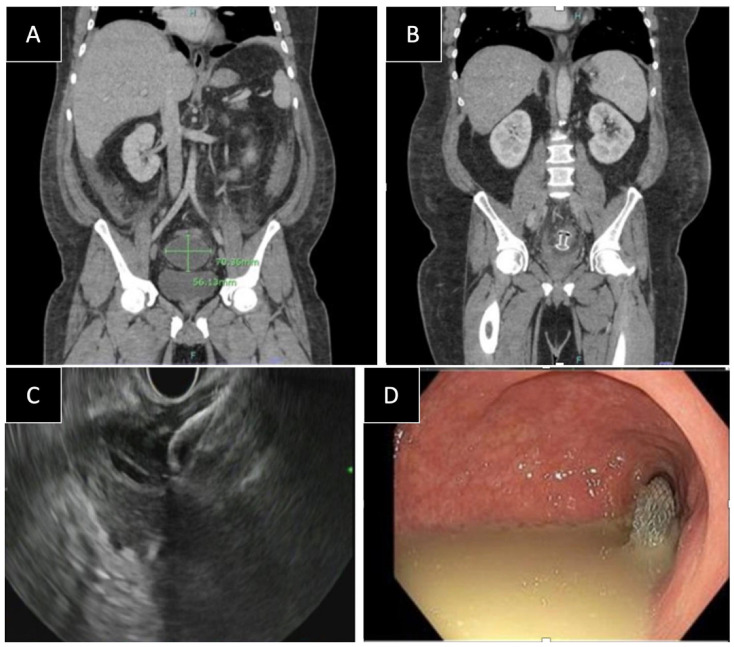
(A) 70 mm × 56 mm pelvic fluid collection. (B) Abdominal-pelvic CT performed 48 h post-LAMS placement with complete resolution of the pelvic fluid collection. (C) Endoscopic ultrasound view of LAMS deployment. (D) Endoscopic view post-LAMS deployment.
